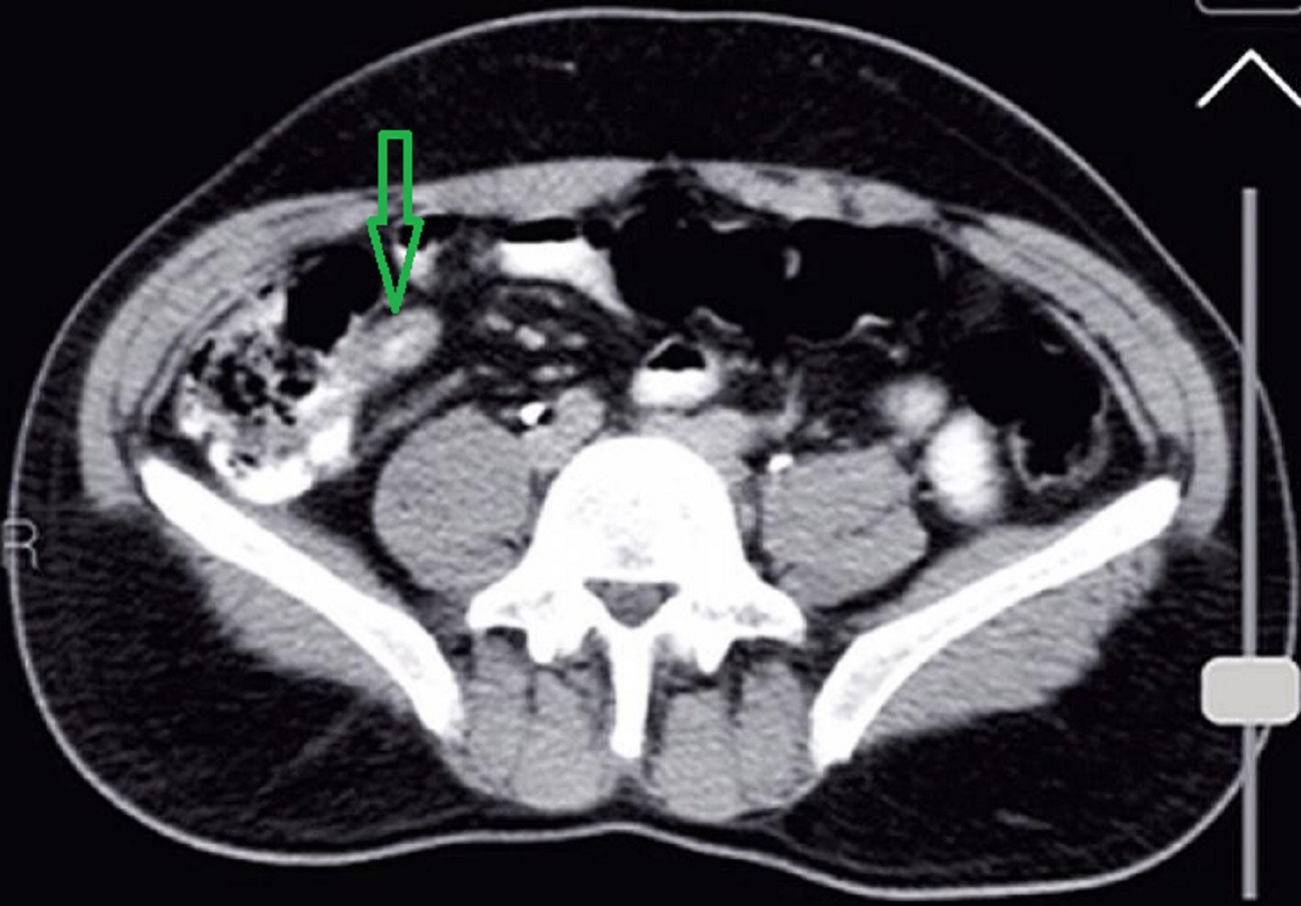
Abdominal CT‐scan, the green arrow shows appendix filled by IV contrast fluid
